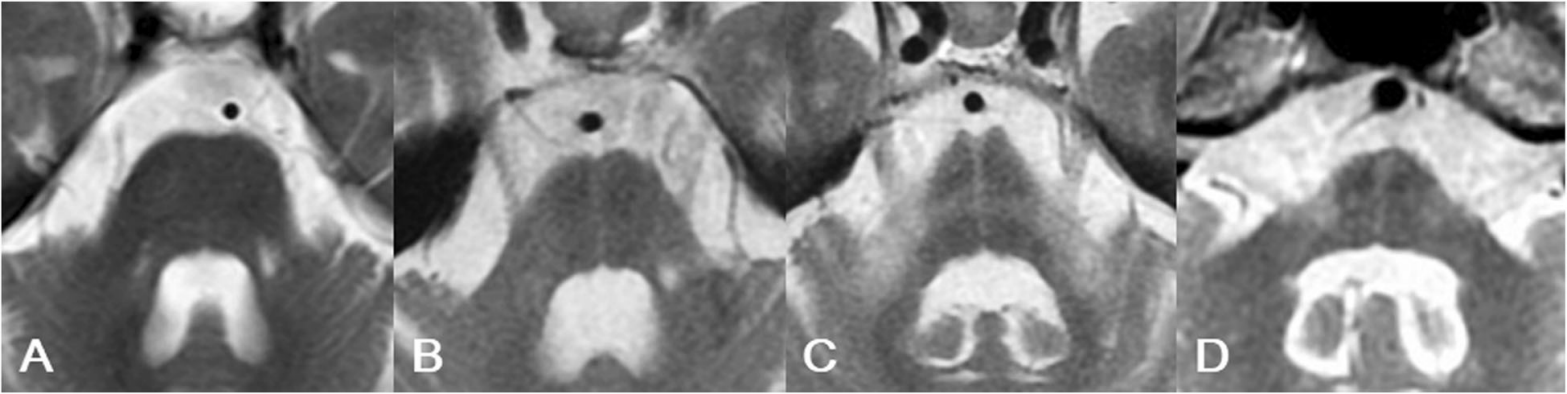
Three grades of the hot cross bun (HCB) sign on magnetic resonance imaging. a Grade 0 (negative) HCB sign in a 74-year-old woman with cerebellar-type multiple system atrophy (MSA-C) with a disease duration of 0.3 years. There is no signal change in the ventral pons. b Grade 1 HCB sign in a 74-year-old woman with MSA-C with a disease duration of 2.3 years. A vertical T2 high-intensity line is clearly seen in the ventral pons. c Grade 2 HCB sign in a 61-year-old man with MSA-C with a disease duration of 2.3 years. Both horizontal and vertical lines are clearly seen in the ventral pons. d Grade 2 HCB sign in a 75-year-old woman with SCA3 with a disease duration of 8.2 years. Both horizontal and vertical lines are clearly seen in the ventral pons
